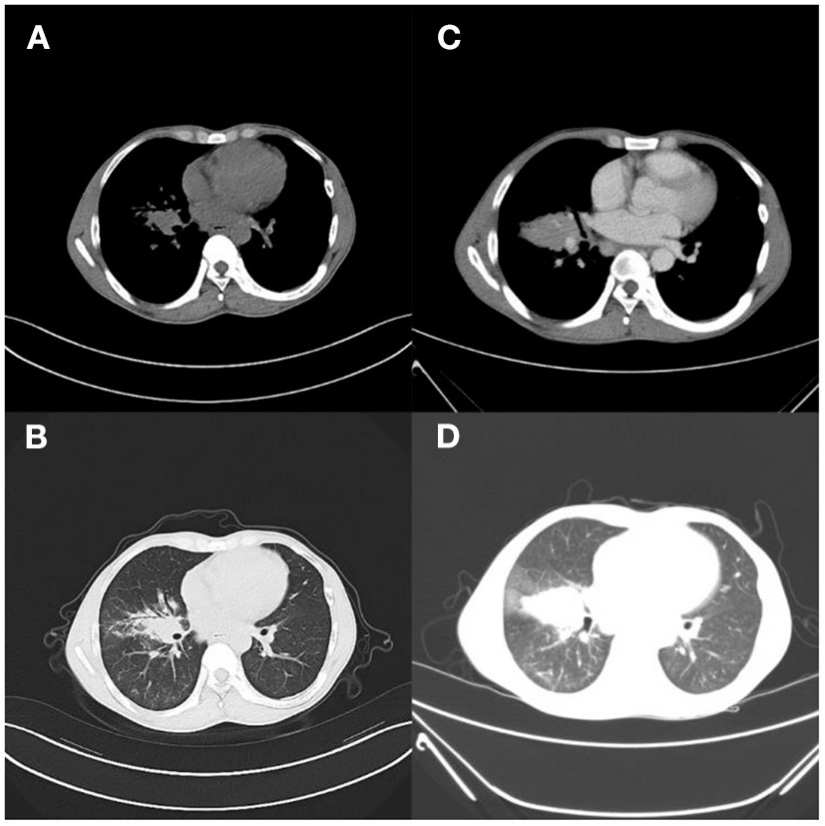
Case 1. (A,B) Outpatient lung CT; (C,D) 9 days after admission, pulmonary inflammation worsened, nodules increased, and lymph nodes were more enlarged.
